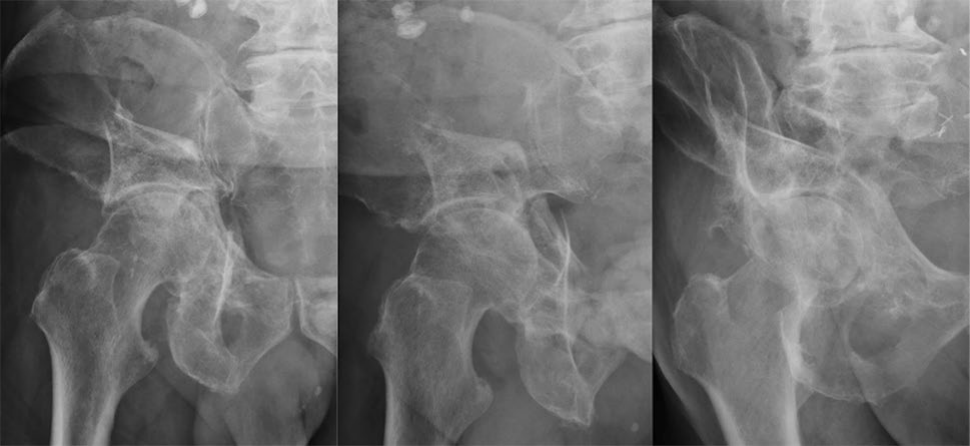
6-month follow-up radiographs following conservative management of elderly patient in Fig. 1: AP and Judet views
